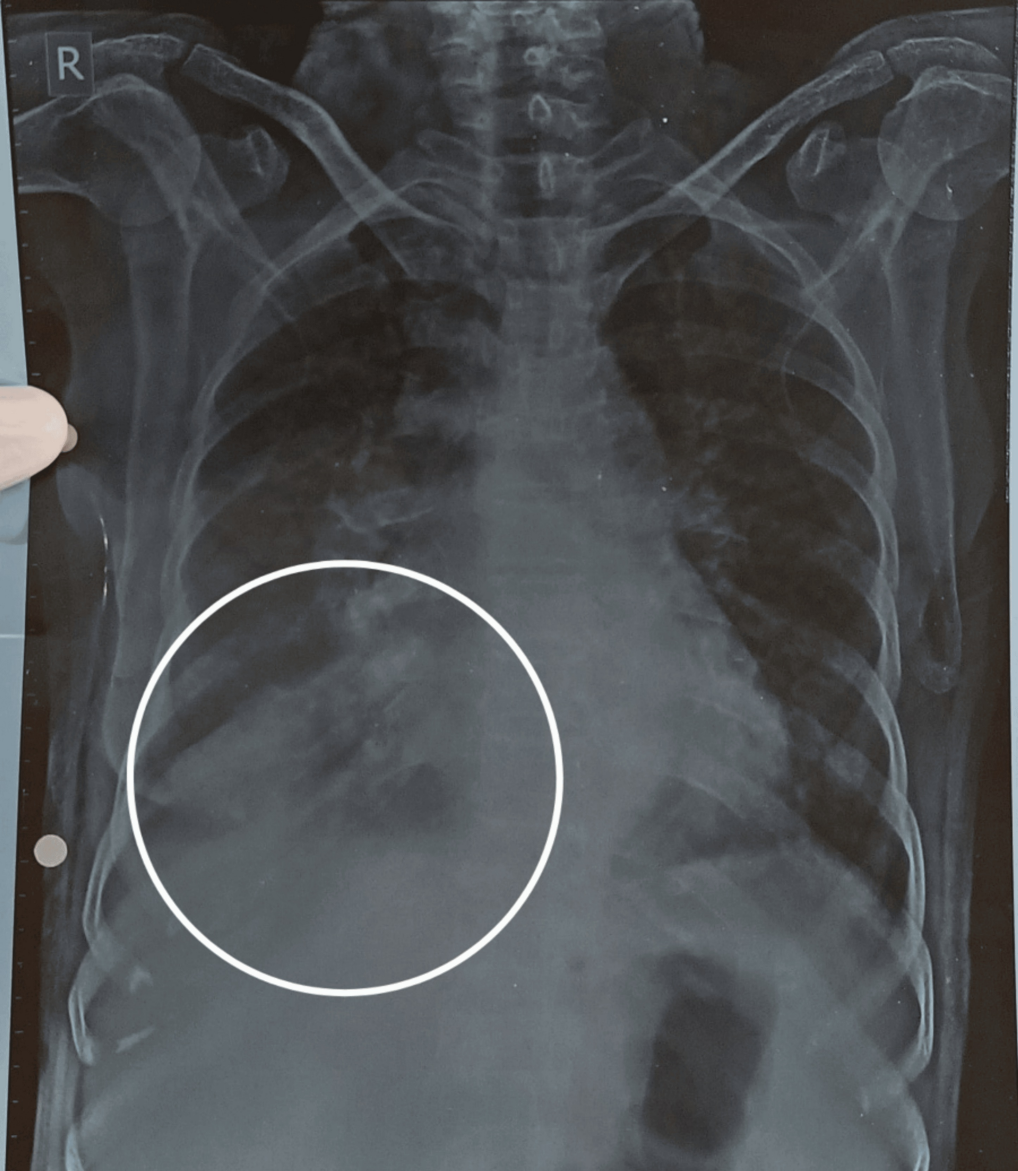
Right-sided chest radiograph PA view showing mild pleural effusion. The circular area depicts increased homogenous density near the costophrenic angle PA: posterior anterior
